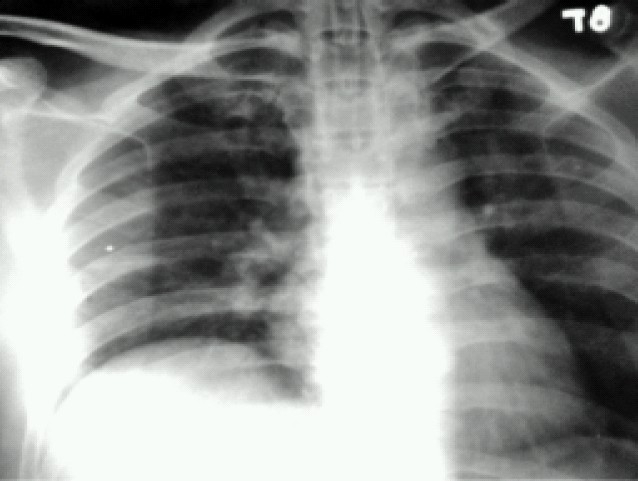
Chest X-ray on 19th day showing clear lung fields
